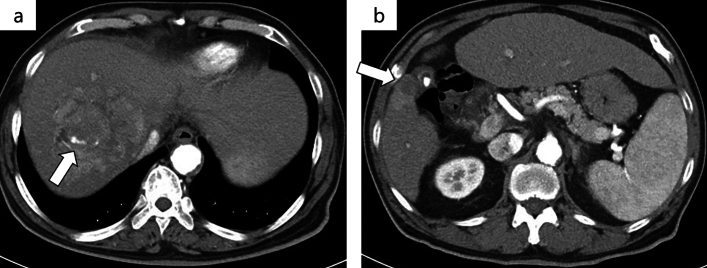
CT after 3 courses of atezo/bev therapy. a The main tumor had intra-tumoral hemorrhage (white arrow). b The other daughter tumors were not contrasted and tended to necrosis (white arrow)
